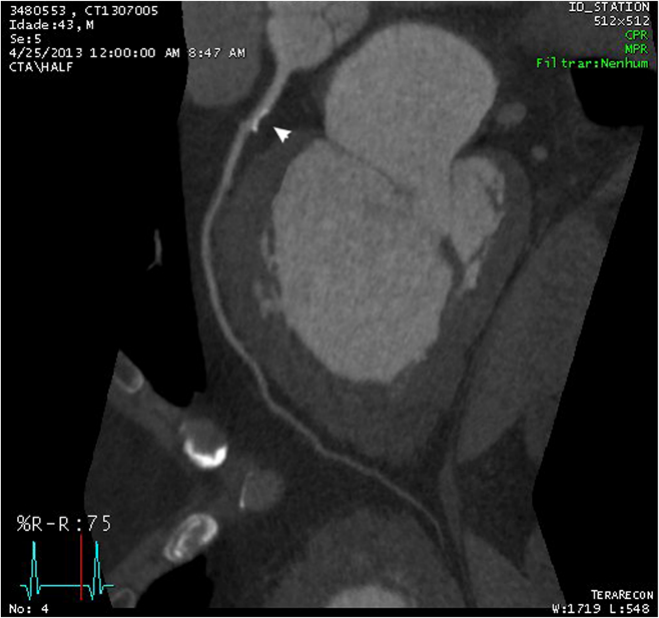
Coronary computed tomography angiogram showing a calcified nonobstructive plaque in the proximal left anterior descending coronary artery.
